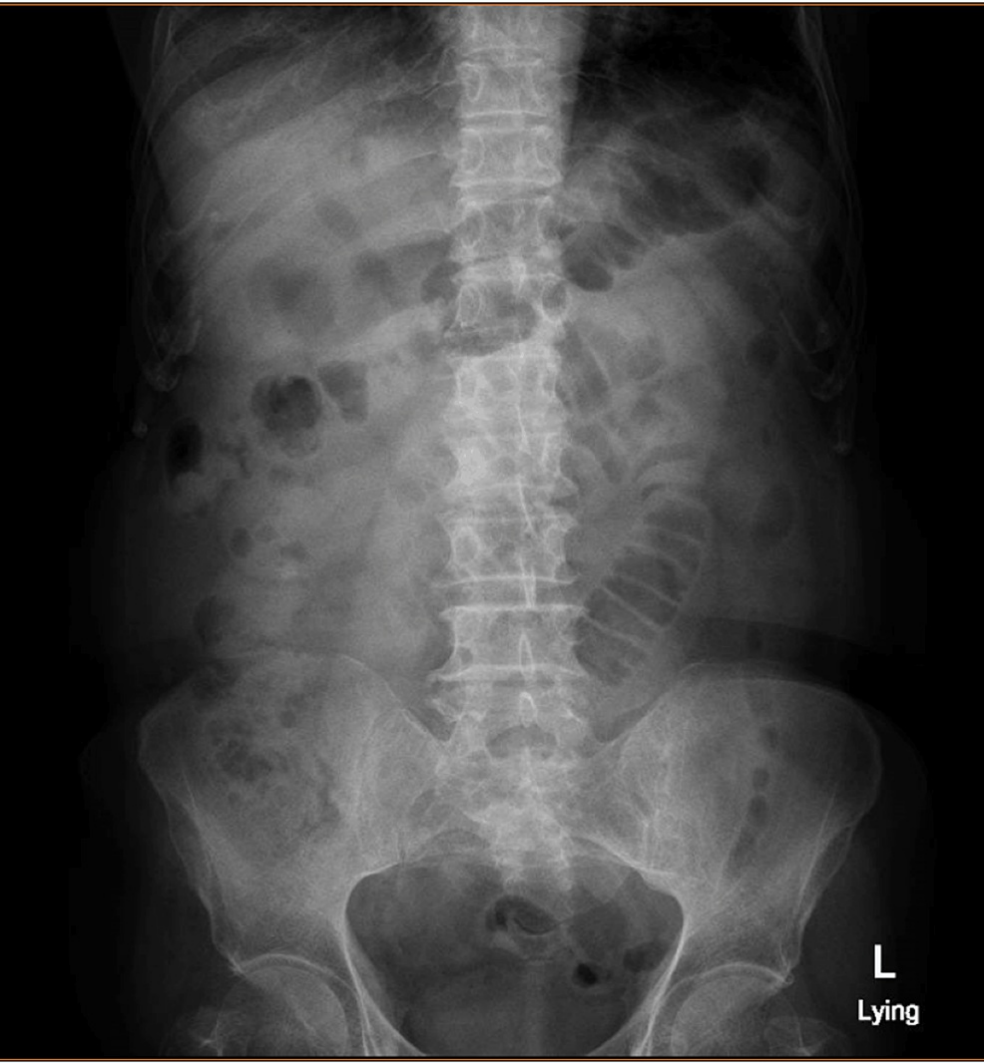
Preoperative abdominal X-ray showed dilated loops with excessive gases.
